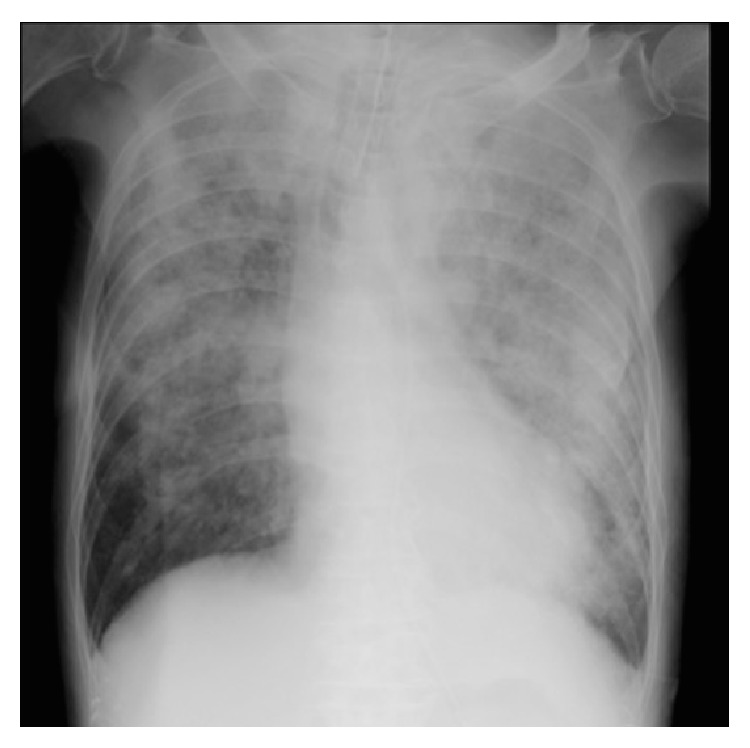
A chest radiograph on admission to the intensive care unit showed diffuse bilateral infiltrates, consistent with pulmonary edema and hemorrhage.
